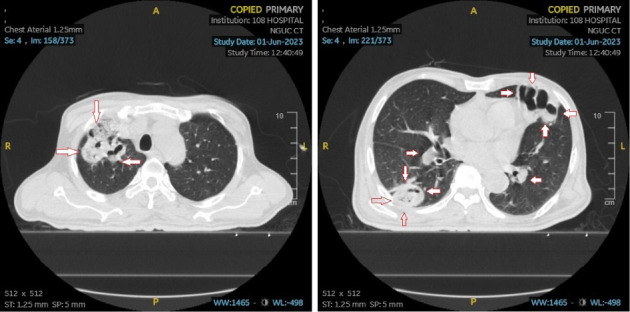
Computed tomography image of the chest shows multiple abscesses in both lungs, the largest abscess size of the right lung is 48 × 50 mm, the largest abscess size of the left lung is 36 × 54 mm.
